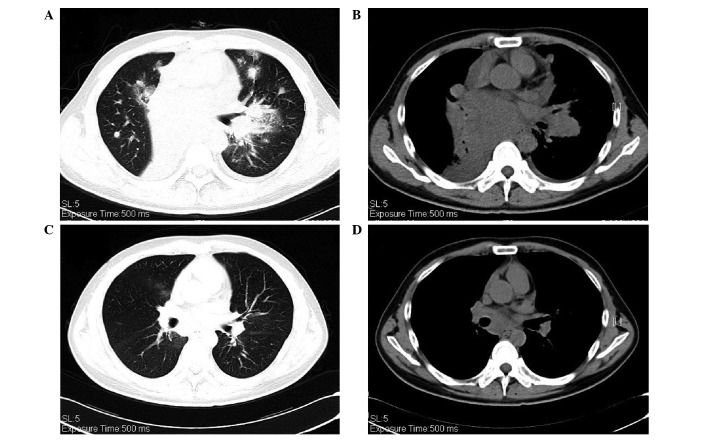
Computed tomography (CT) of the chest to observe the response to crizotinib treatment. CT scans of the chest were obtained (A and B) at baseline and (C and D) following 4 weeks of crizotinib treatment.
